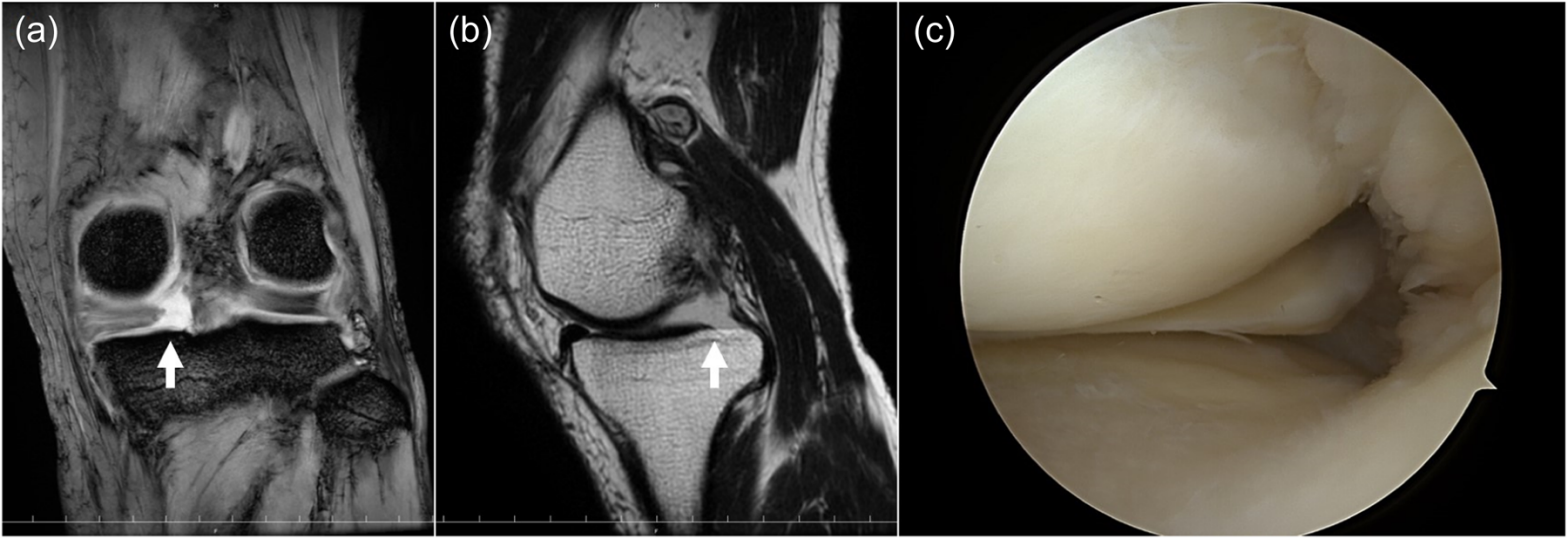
MMPRTs MRI findings of MMPRT are shown. Giraffe neck sign in coronal view (a) and ghost sign in sagittal view (b) are shown. Also, arthroscopy revealed discontinuity of MMPRT (c). MMPRT, medial meniscus posterior root tear; MRI, magnetic resonance imaging.
